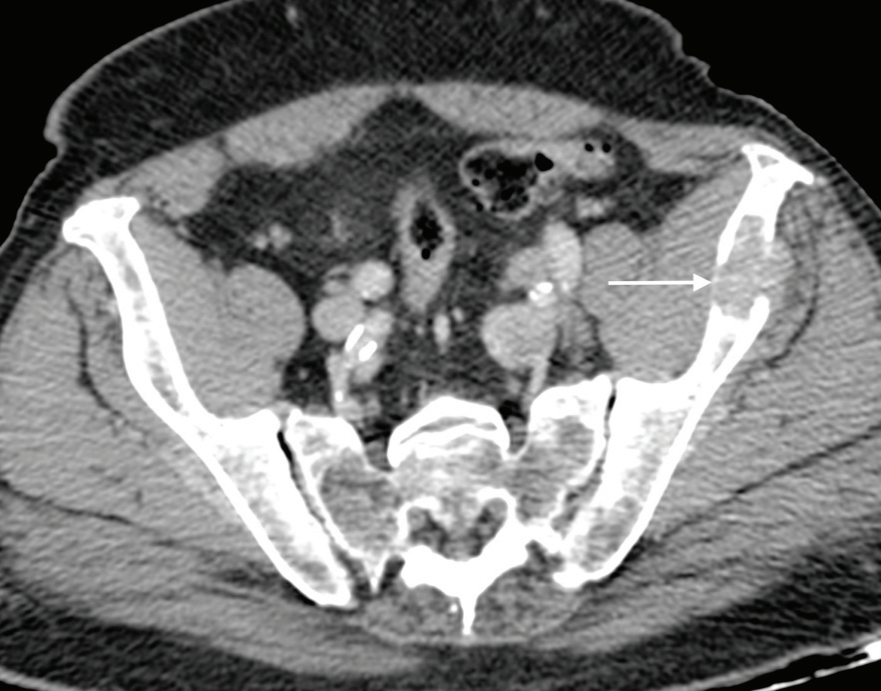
Axial post contrast CT of the pelvis shows a lytic left iliac wing metastatic deposit (arrow) in an 84-year-old man with long-standing bone metastases in castration-resistant metastatic prostate carcinoma.
